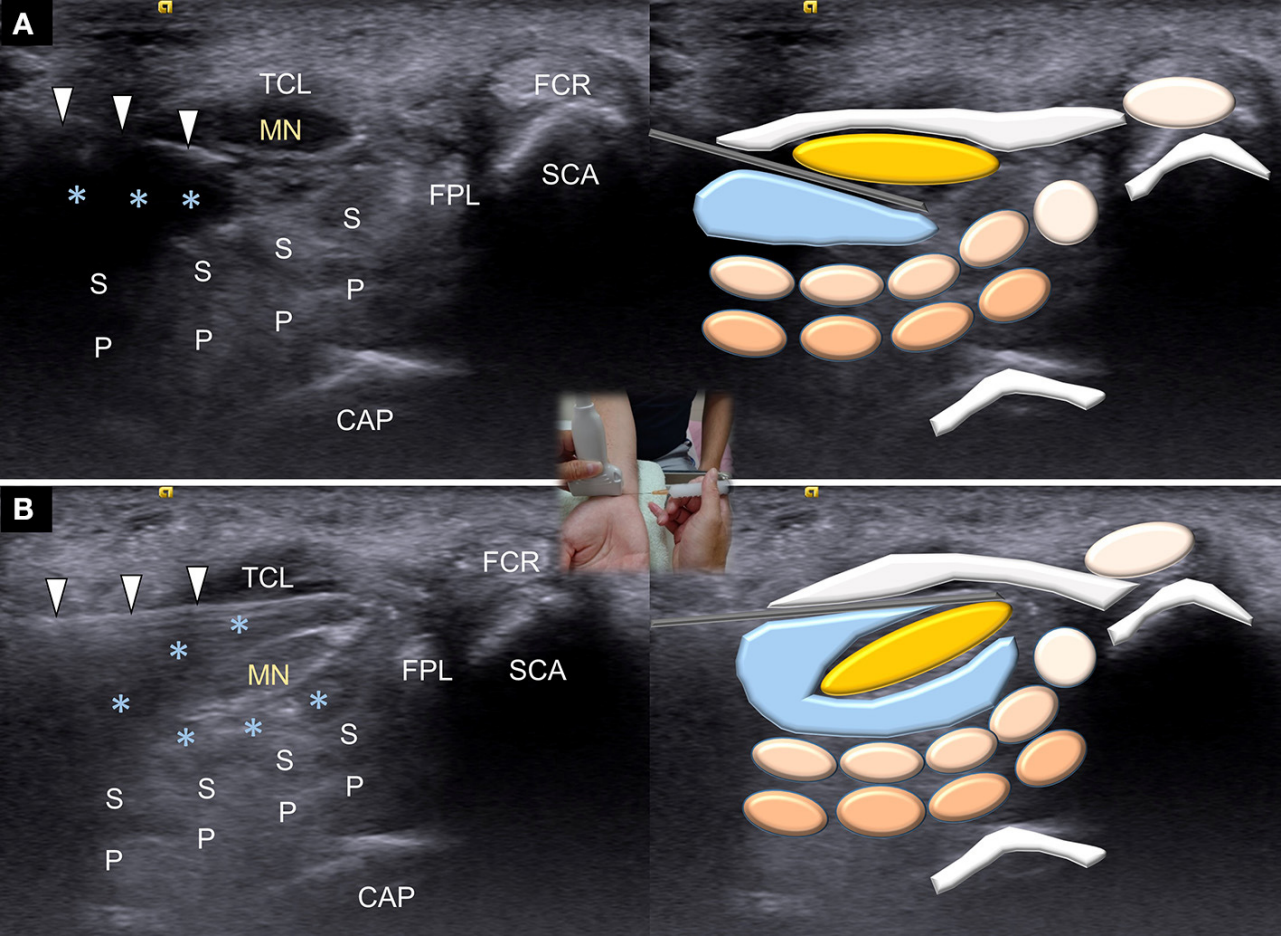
Ultrasound guided hydrodissection for the surface under (A) and above (B) the median nerve. MN, median nerve; asterisks, injectate; TCR, transverse carpal ligament; FCR, flexor carpi radialis tendon; FPL, flexor pollicis longus tendon; S, flexor digitorum superficialis tendon; P, flexor digitorum profundus tendon; SCA, scaphoid bone; CAP, captitate bone.
